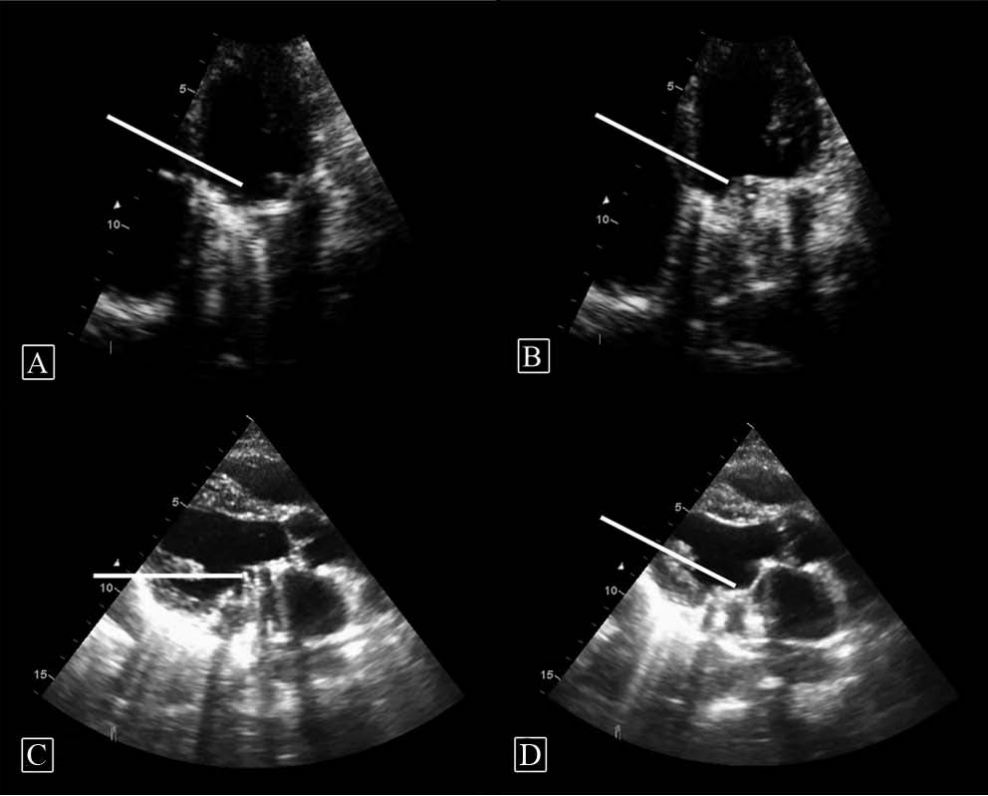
Transthoracic echocardiography (TTE). Appearance at 24 hours post treatment (A and B) showing (with arrows) both mitral leaflets to be reasonably mobile: (A) in open position; (B) in closed position. (C and D) TEE appearance at 48 hours post treatment showing (with arrows) fully mobile both mitral leaflets: (C) in open position; (D) in closed position.
